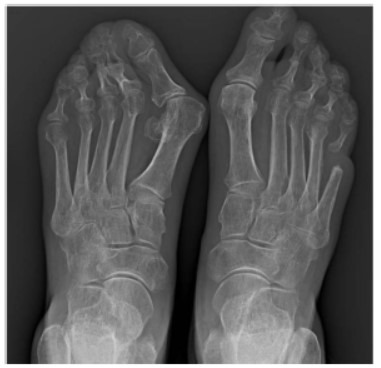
Radiographic evaluation nine months after the initial presentation, demonstrating detachment of the two stumps of the fifth ray of the right foot. Taken on September, 2024.
